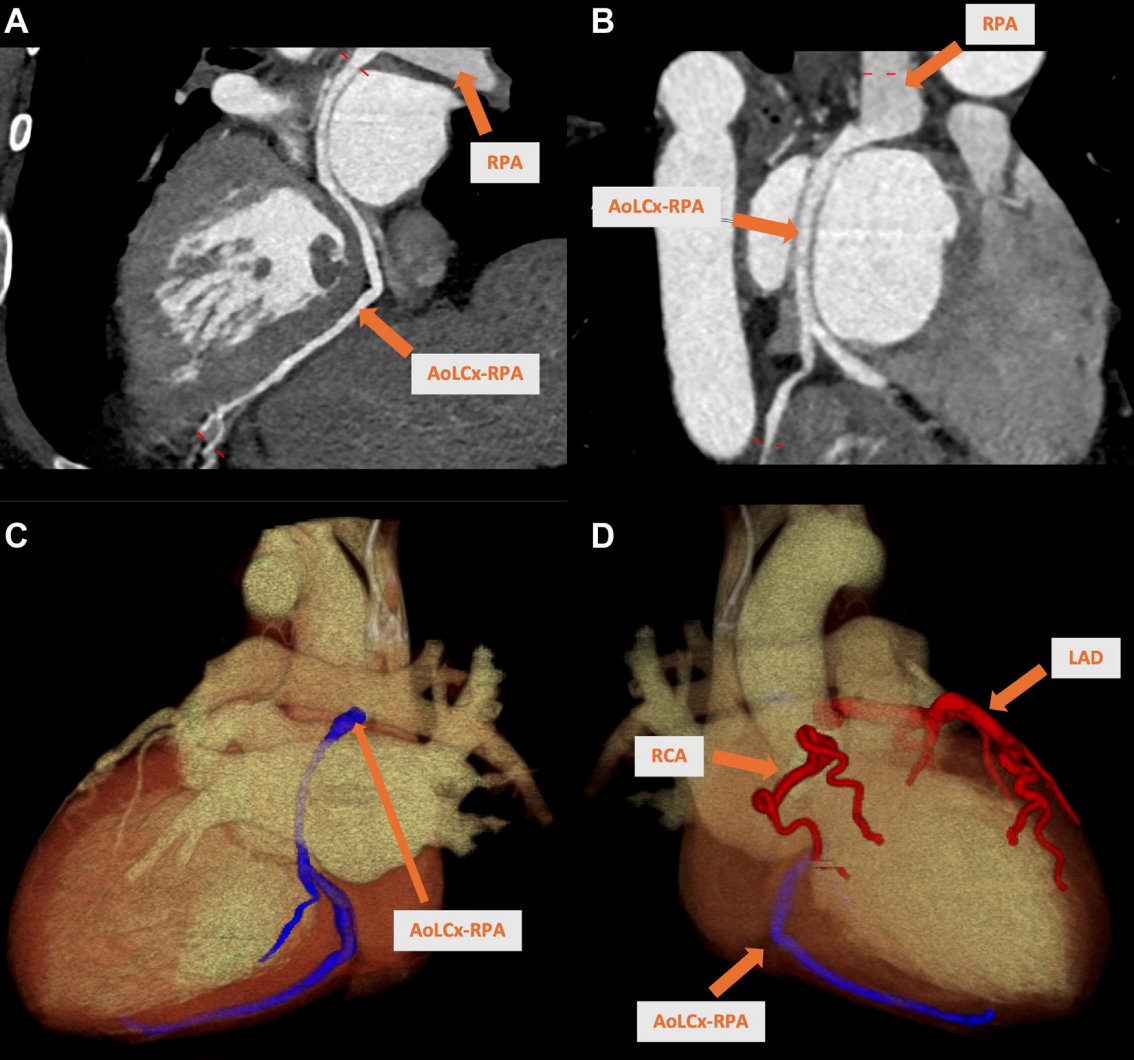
Cardiac CT Images Cardiac computed tomography images (A and B) and 3-dimensional segmentation (C and D) show anomalous origin of the LCx from the right PA, approximately 2.3 cm from the PA bifurcation, with multiple fistulous communications to the LAD and RCA. LAD and RCA have normal aortic origins, with the LAD appearing prominently tortuous (up to 8 mm proximally) and the proximal RCA measuring up to 6 mm. No coronary plaque or stenosis is observed. AoLCx-RPA = anomalous left circumflex artery from the right pulmonary artery; LAD = left anterior descending artery; PA = pulmonary artery; RCA = right coronary artery.
